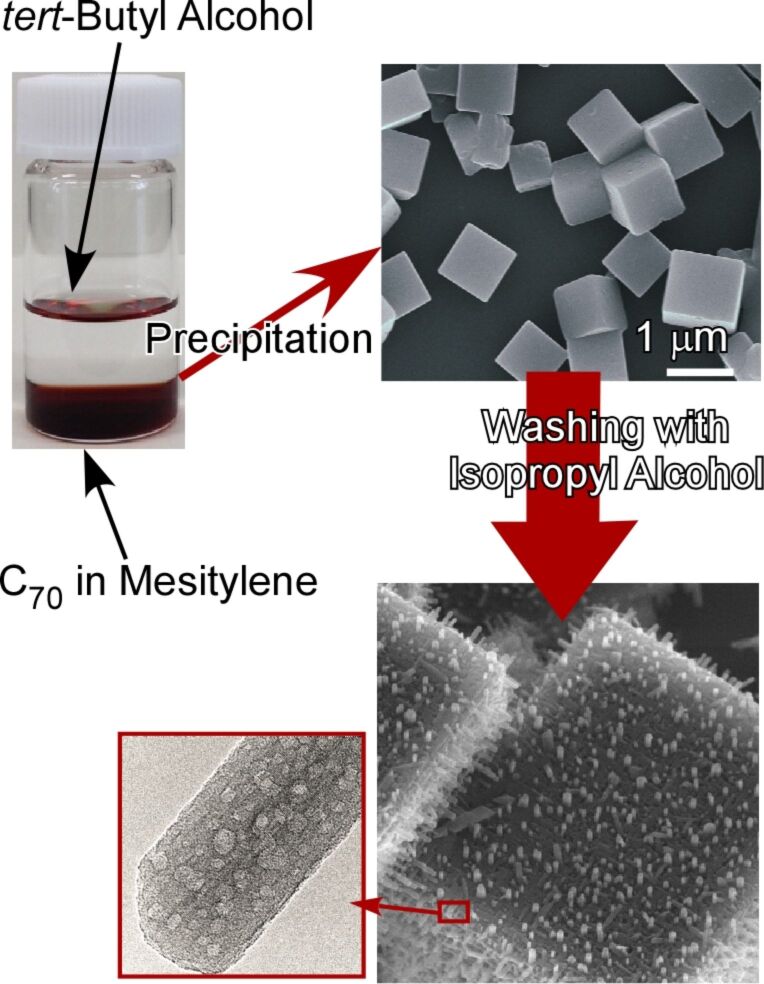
Three-dimensional cubic structures can be fabricated from C_70_ molecules through an ultrasound-assisted liquid–liquid interfacial precipitation from *tert*-butyl alcohol and mesitylene. The formed cubes can be further transformed into needle-on-cube (one-dimensional structure on the surface of three-dimensional object) structures simply by washing with isopropyl alcohol at room temperature. Reproduced from [75], copyright 2019 The authors.
